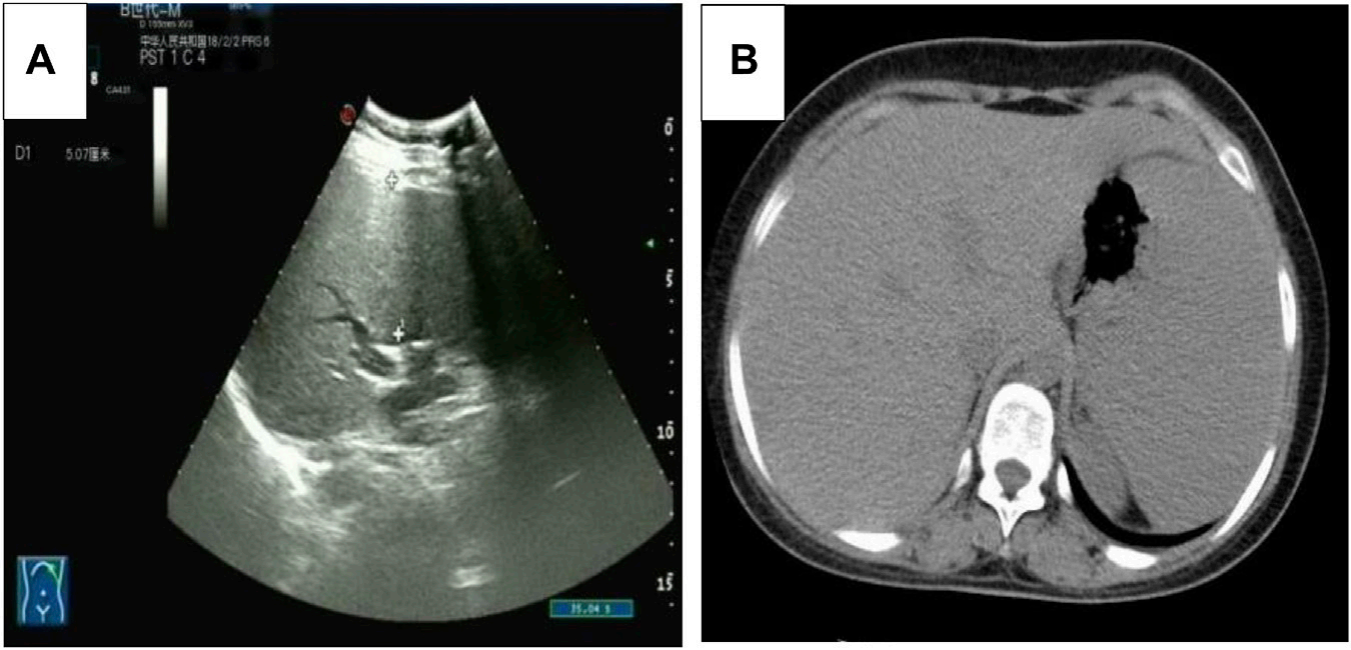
(A) The abdominal ultrasound image of the patient. (B) The axial abdominal CT image of the patient.
